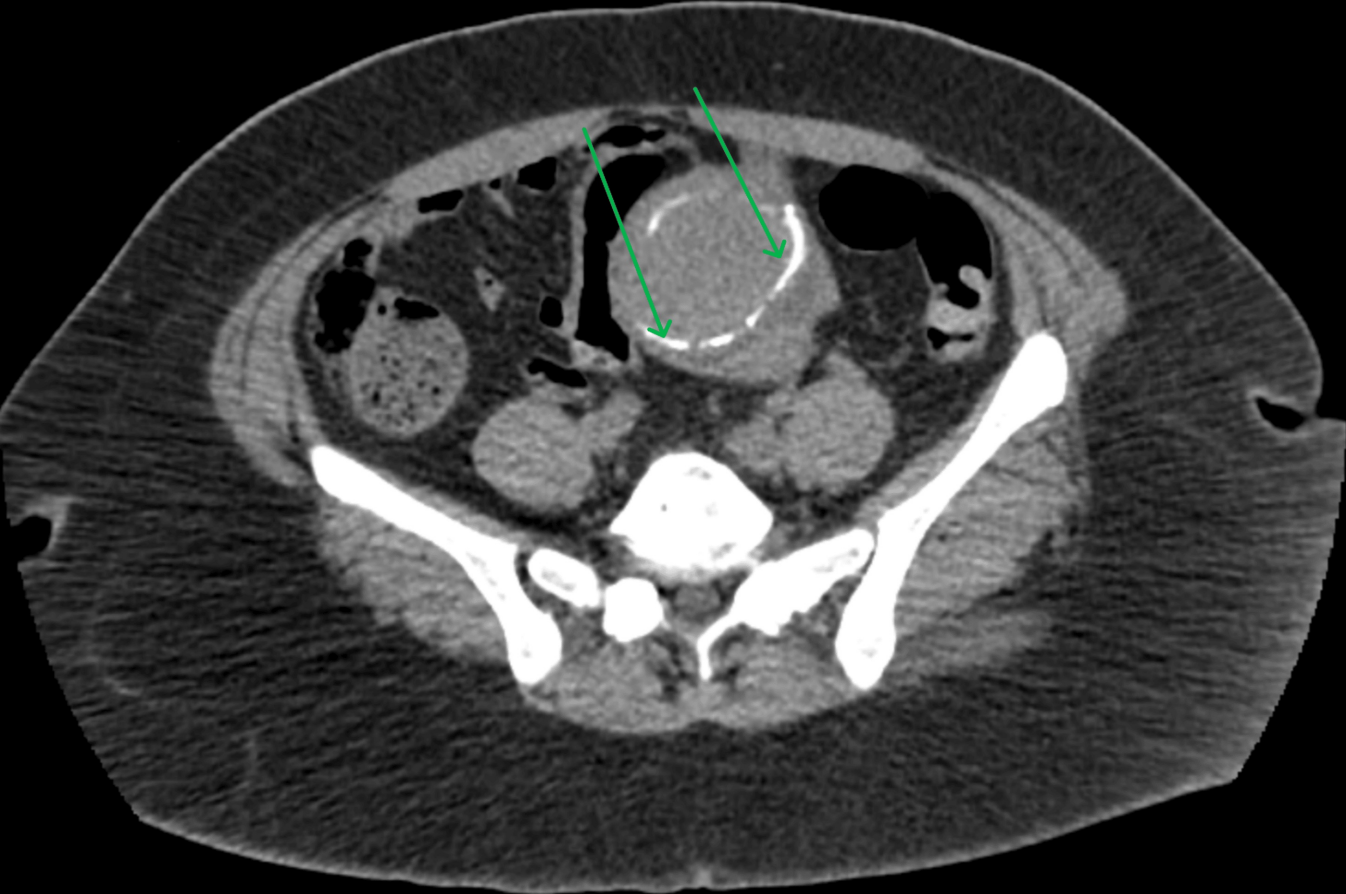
Abdomino-pelvic CT scan 2 Axial abdominopelvic CT scan at the level of the uterus. The image demonstrates a rim of calcified fibroid within the uterine wall, indicative of a degenerating leiomyoma. No evidence of bowel obstruction, perforation, or abscess.
